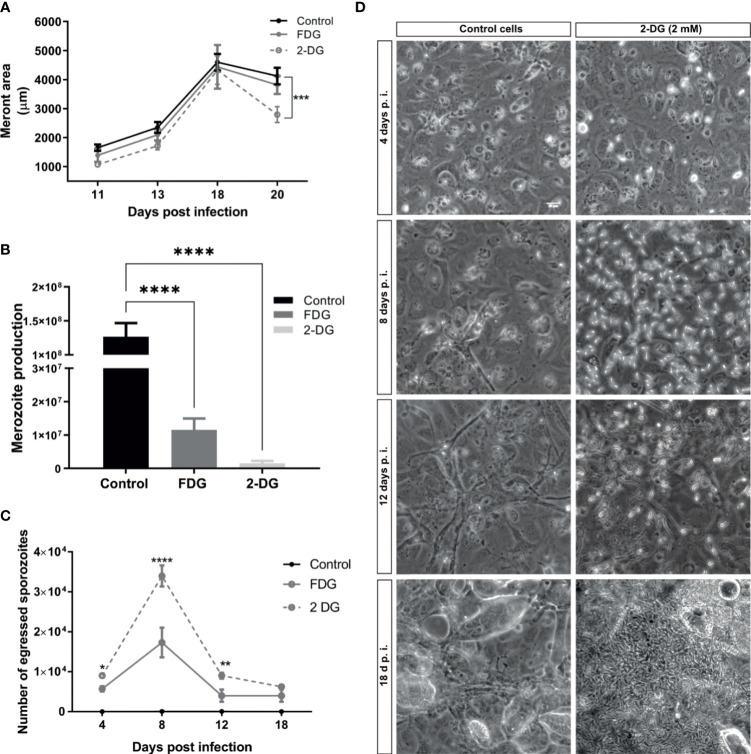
2-DG and FDG treatments diminish merozoite I production and induce *E. bovis* sporozoites egress. (A)
